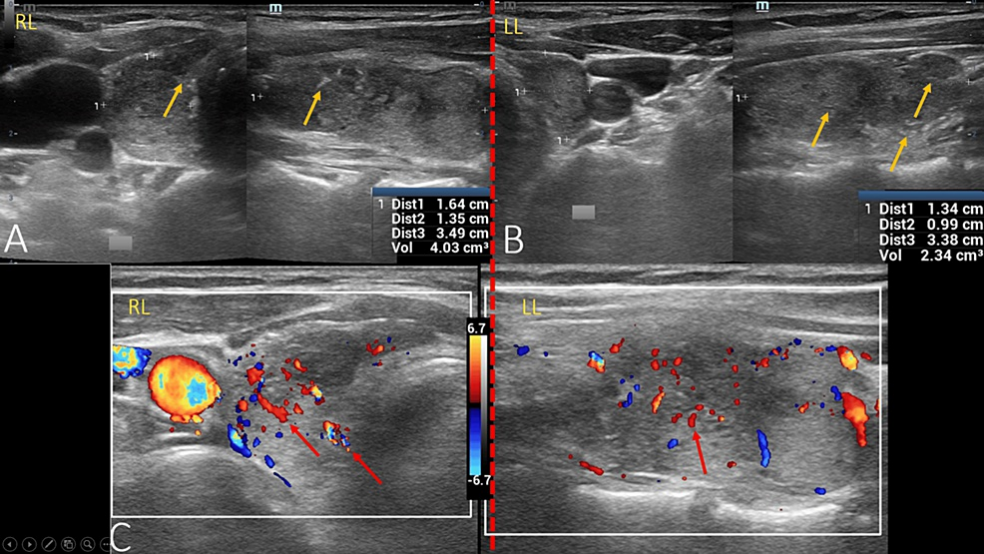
Thyroid ultrasound images A: atrophic right thyroid lobe (RL), heterogeneous hypoechoic echostructure with a few hyperechoic septa, producing a pseudo-nodular appearance (yellow arrows). B: atrophic left thyroid lobe (LL), with heterogeneous hypoechoic echostructure due to the presence of echogenic punctuations (yellow arrows). C: hypervascularization aspect on color Doppler of both thyroid lobes (red arrows).
